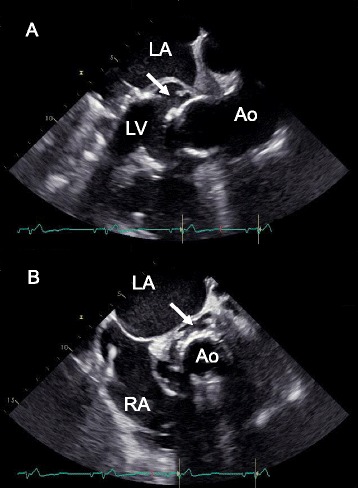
Transesophageal echocardiography (TEE) findings on cardiological follow-up evaluation 06/2014 after urgent prosthetic mitral valve replacement was successfully performed in 11/2013. In the different views, A and B, the abscess formation (*arrows*) around the mechanical valved conduit is shown, which has direct contact to the left ventricle (LV). LA denotes for the left atrium, RA right atrium and Ao aorta ascendens
